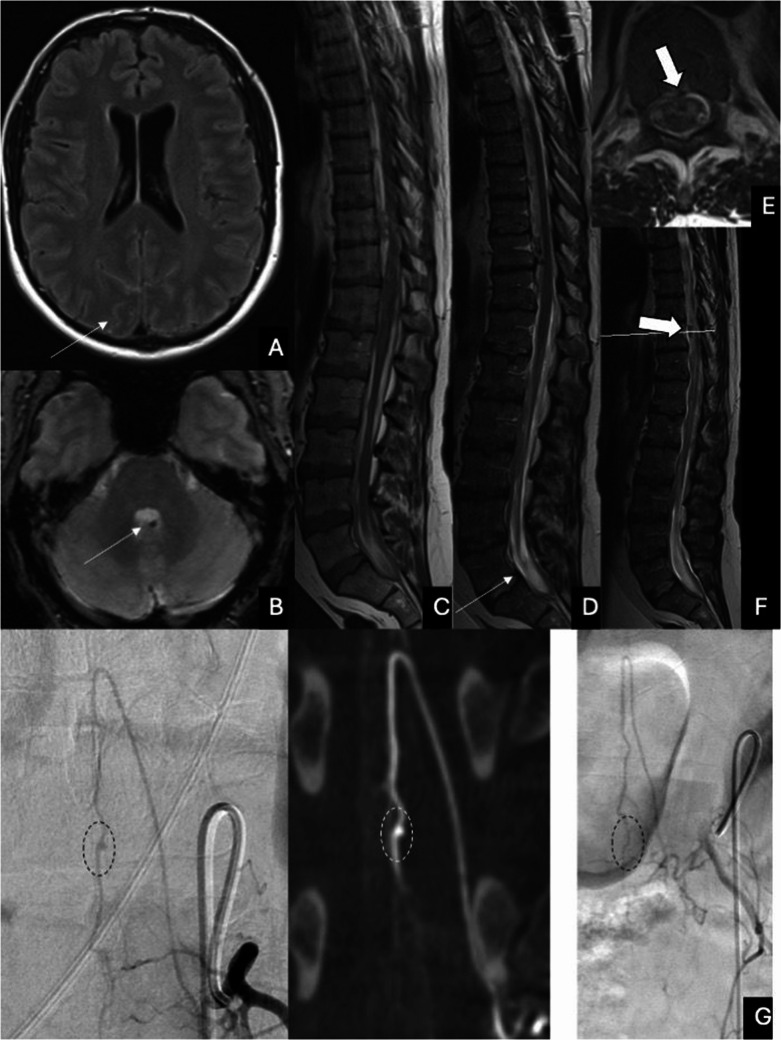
Representative case (Patient 4). On cranial MRI, cerebral SAH is seen on FLAIR (A) and T2* (B) images. On spinal MRI, spinal SAH is seen in the thecal sac (C and D), and a small cystic lesion with flow void in the ventral dural sac at T11 level (E and F). DSA shows a fusiform aneurysm of the ASA at T12 level (G)
